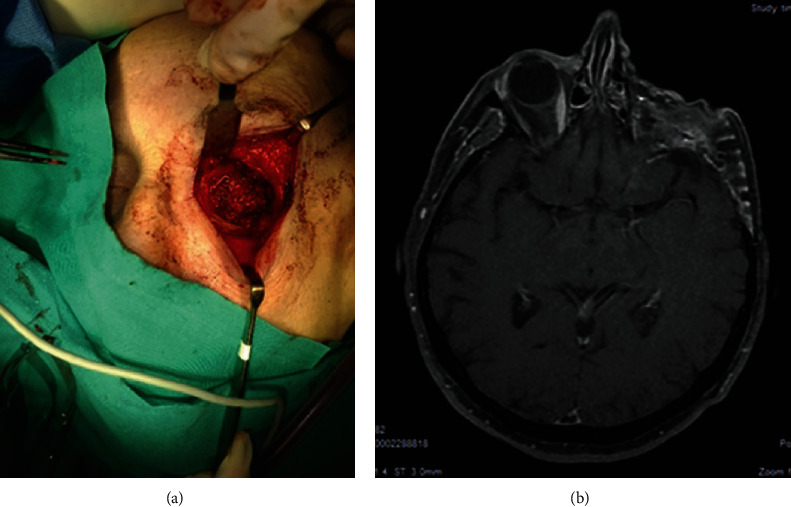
Case 4. (a) Photograph of surgical exenteration. Temporal muscle fills the posterior part of the right orbit. (b) Axial view MRI postexenteration.
